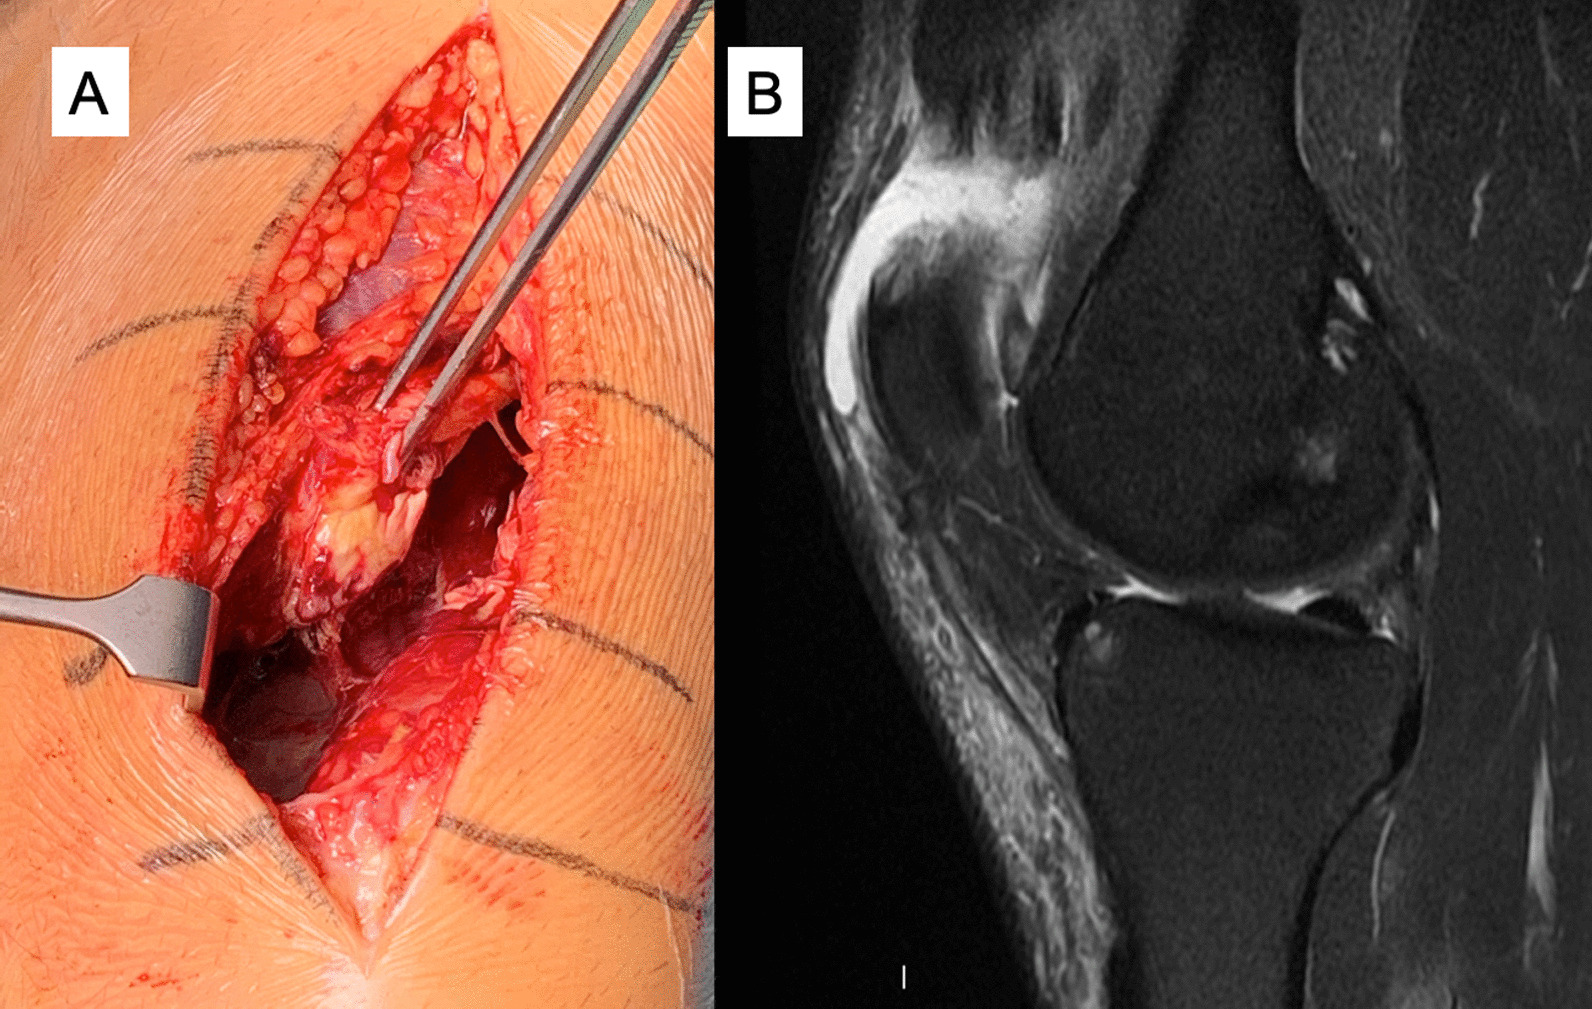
Quadriceps tendon complete rupture: operative image (A) and sagittal proton density fat saturated MRI image (B)
